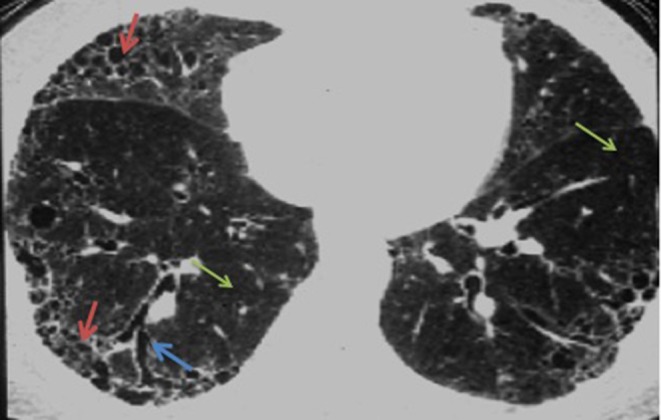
HRCT of a usual interstitial pneumonia (UIP) pattern, characterized by honeycombing (red arrows), and traction bronchiectasis (blue arrow). Normal lung tissue is signaled with green arrows.
